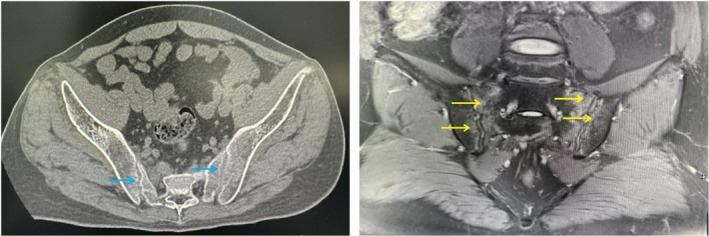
SIJ axial CT scan showed rough edges of the bilateral sacroiliac joints (blue arrow, left panel). SIJ coronal MRI scan showed bone marrow edema in the sacral and iliac regions, especially in the sacral region (yellow arrow, right panel).
